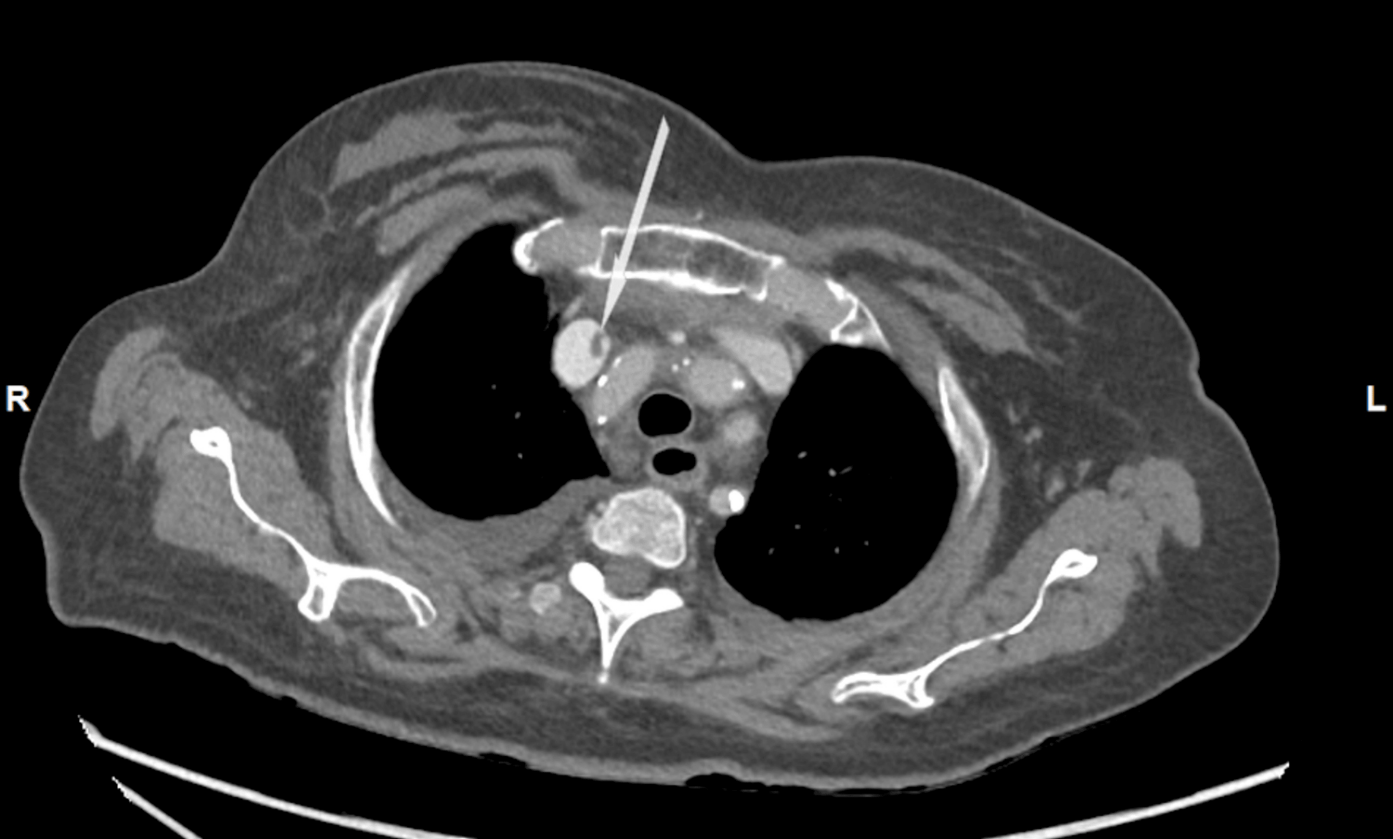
CT thorax, abdomen and pelvis, axial view (Case 3). Arrow shows thrombus in the superior vena cava. Bilateral small pleural effusions present.
